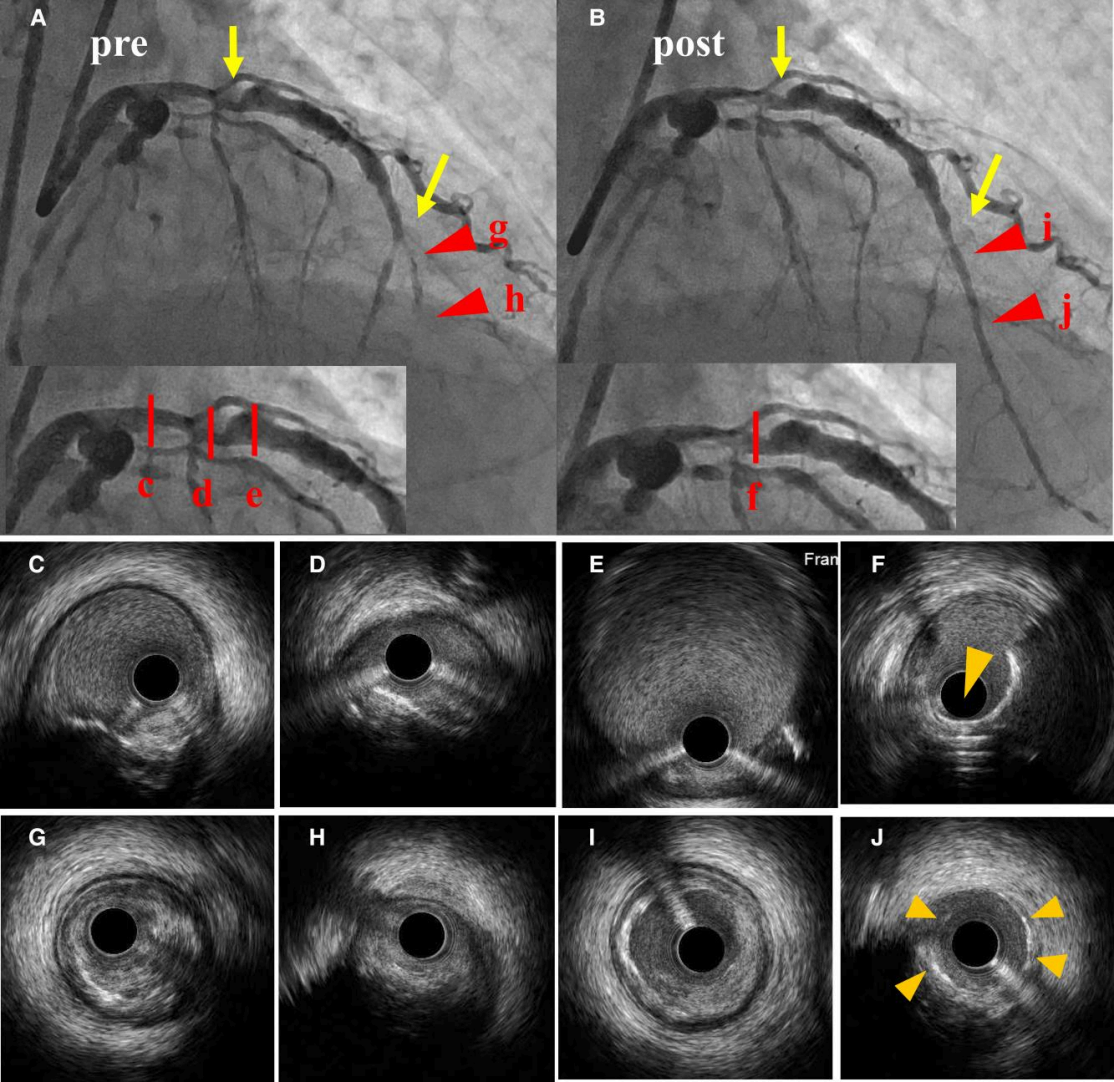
Percutaneous coronary intervention in the left anterior descending artery. Percutaneous coronary intervention (PCI). Initial (*A*) and final angiography after PCI (*B*) are shown. The target lesions are indicated by arrows. Intravascular ultrasound (IVUS) images reveal the proximal portion of the stenosis (*C*), proximal target stenosis with calcification (*D*), and the distal coronary aneurysm (*E*). (*F*) The final IVUS image after rotablation with a 2.0 mm burr (175 000 rpm) followed by drug-coated balloon dilatation, shows that the calcification has been effectively removed (arrowheads). (*G*), (*H*) The distal lesion before PCI. (*I*), (*J*) The final IVUS image after rotablation with a 1.5 mm burr (175 000 rpm) followed by drug-coated balloon dilatation, shows that the calcification and the hard fibrous plaque have been ablated. Image of a vessel wall with drug application (arrowheads).
